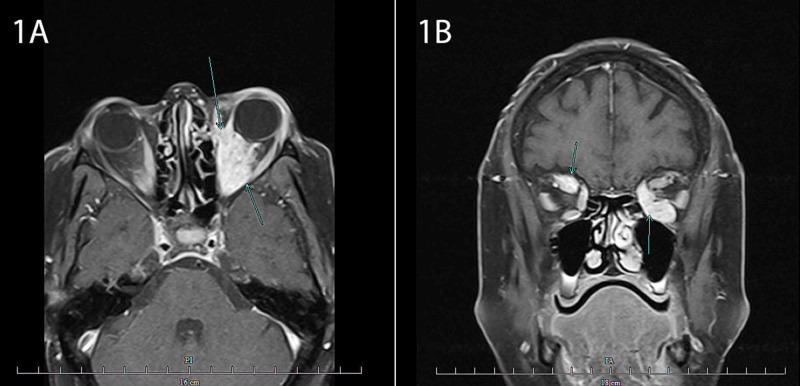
Orbital Magnetic Resonance Imaging 1A: Axial MRI of the orbits with contrast revealing a left mass lesion (blue arrows). 1B: Coronal MRI of the orbits with contrast revealing a left soft tissue mass engulfing the medial and inferior extraocular muscles and a right soft tissue mass involving the superior rectus muscle (blue arrows). MRI: Magnetic Resonance Imaging
